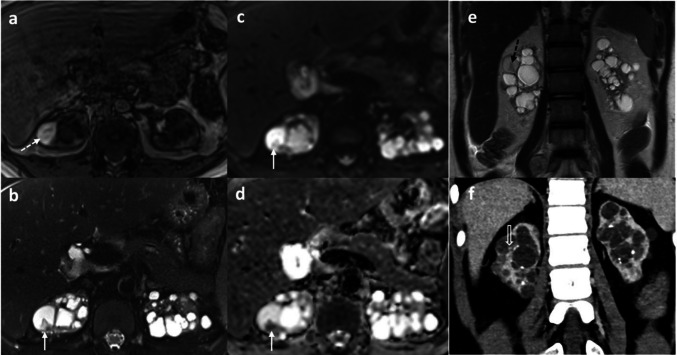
Plain MR abdomen screening in a 15-year-old boy with acquired cystic kidney disease on long-term peritoneal dialysis. a–d Axial plain MR abdomen shows one of the cysts has high signal (dashed white arrow) on T1-weighted sequence (a), and an intracystic nodule with low signal on T2-weighted sequence (b) and restricted diffusion (c, d), suspicious of malignant transformation. e Coronal plain MR abdomen shows the same intracystic nodule. Iodinated contrast was used instead of gadolinium-based contrast agent to further evaluate the lesion. f Nodular enhancement within the cyst was observed on contrast-enhanced CT. Pathology revealed papillary adenoma
